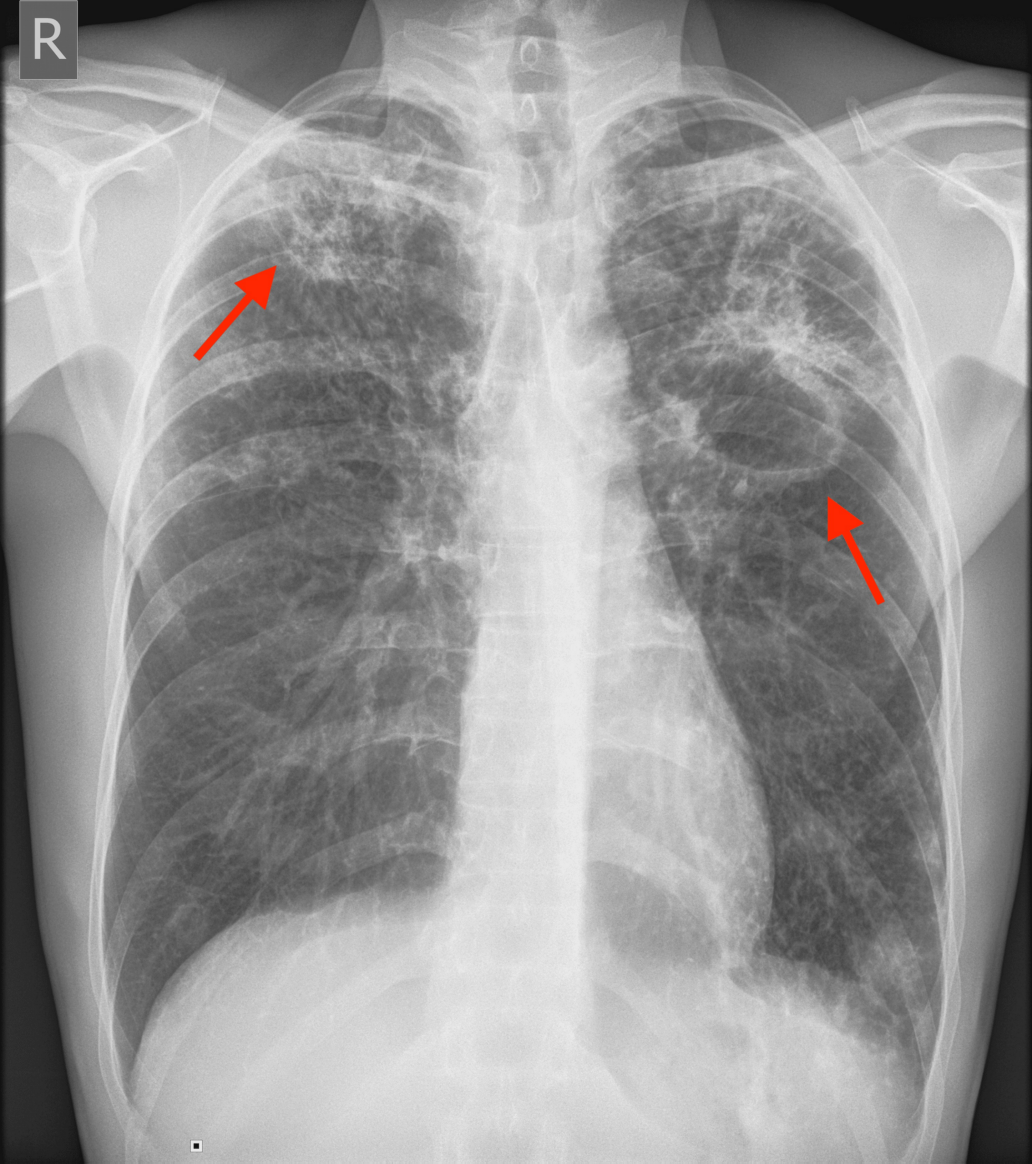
Anterior-posterior view chest X-ray showing bilateral cavernous changes (arrows).
